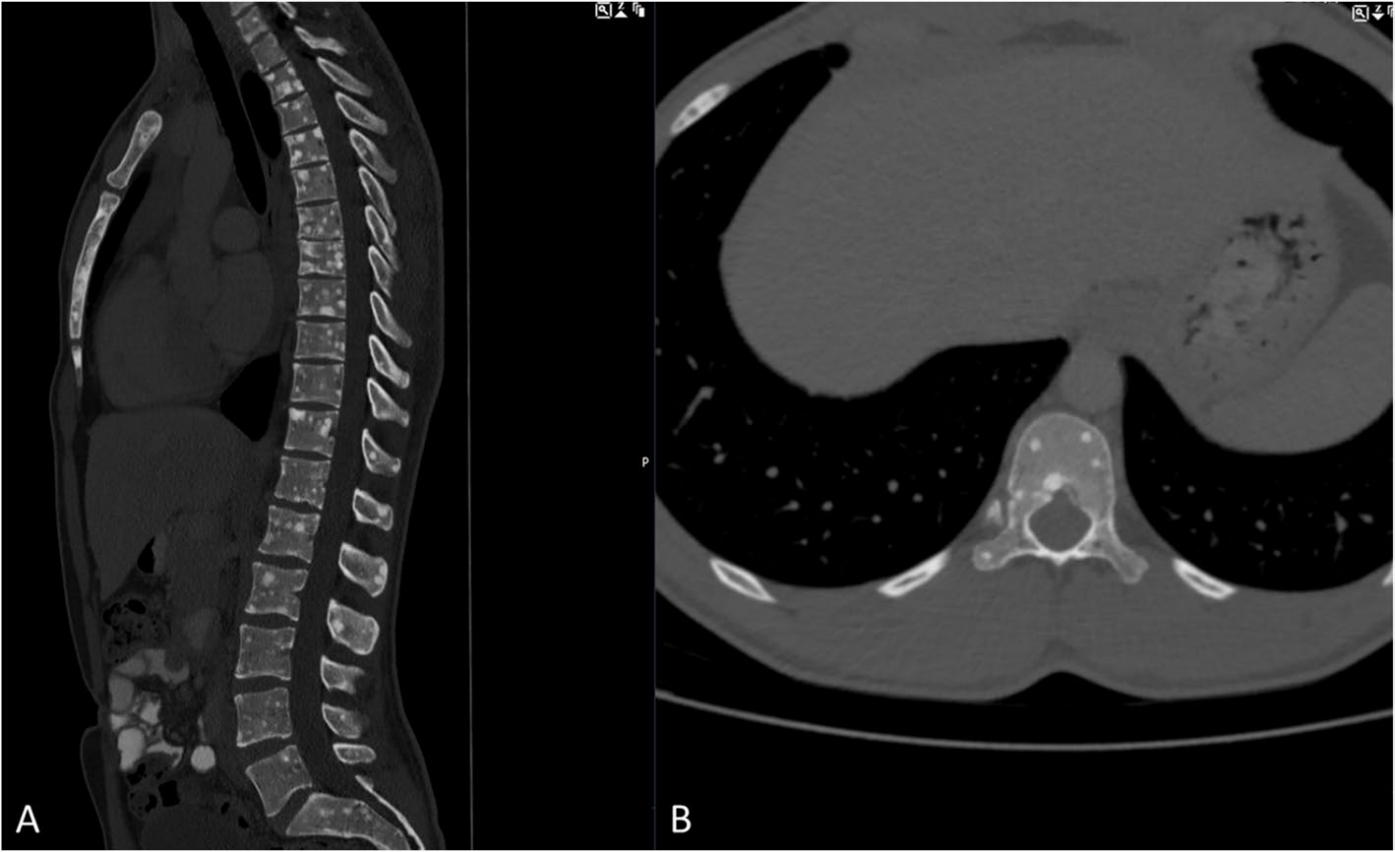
CT images sagittal (A) and axial (B) of the vertebral column demonstrate diffuse intraosseous lesions along the entire spine (cervical to sacral) and the sternum
